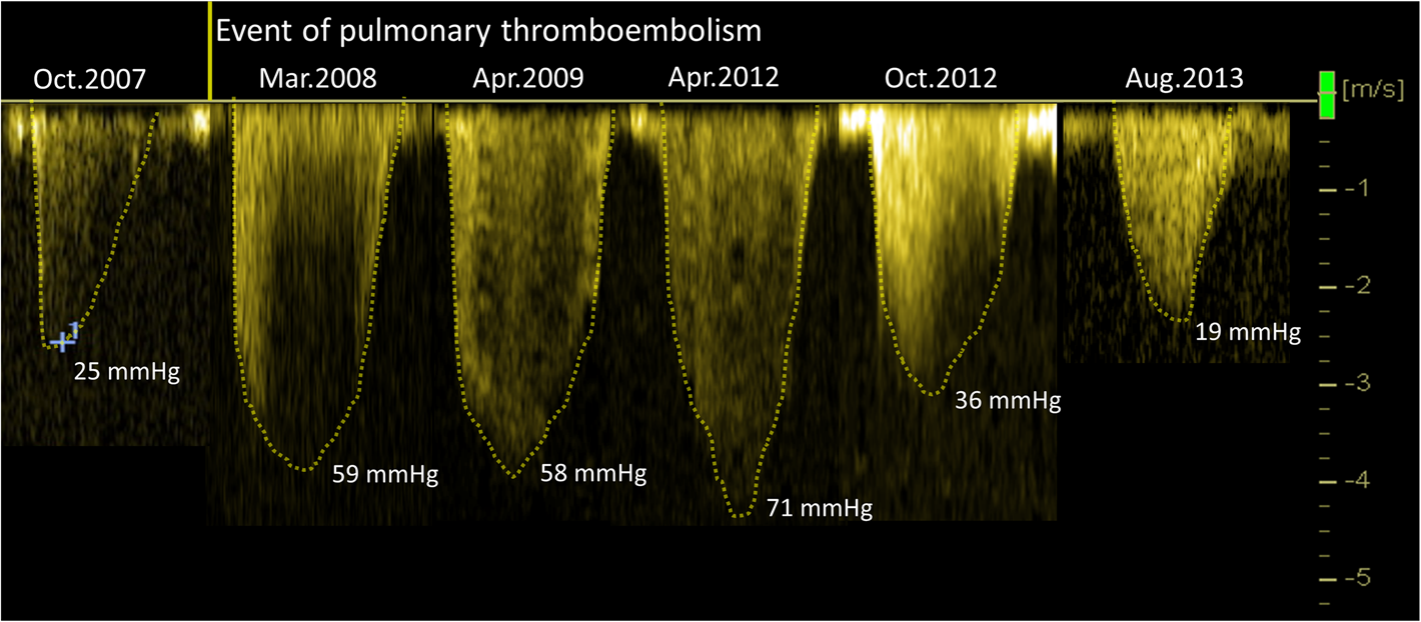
Serial echocardiographic observations of tricuspid regurgitant jet (continuous-wave Doppler recording). Note the pseudo-normal trans-tricuspid pressure gradient (19 mmHg) with the pathological late-peaking velocity spectrum at the final examination (August 2013), clearly distinct from the normal pressure gradient exhibiting an early-peaking velocity spectrum at the examination in 2007, that is, prior to the clinical event of pulmonary thromboembolism.
